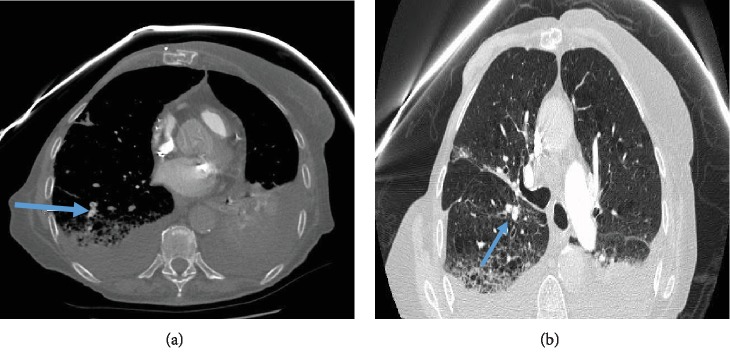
(a, b) A CT angiogram of the chest shows filling defects in the subsegmental arteries (arrows).
